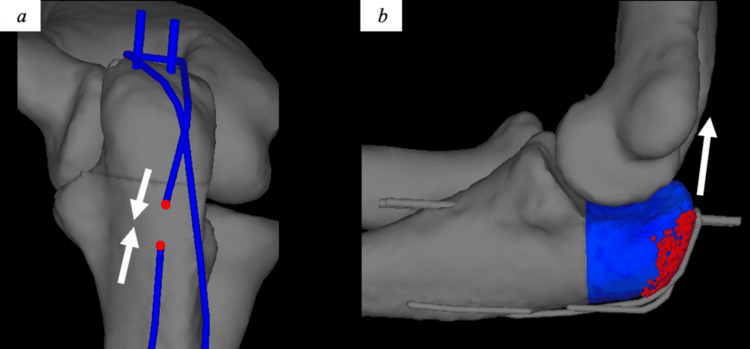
Tension band model created based on 3D CT (a) Blue represents the 2-mm K-wire and 1-mm soft steel wire. The end of the soft steel wire shown in red was pulled in the direction of the arrow with a force of 30 N and maintained. (b) Blue represents the olecranon fragment, and the red dot indicates the triceps brachii attachment. After tightening the soft steel wire, the olecranon fragment was pulled in the direction of the white arrow 3D: three dimensional Image credit: This is an original image created by the author Akitoshi Sakuma
